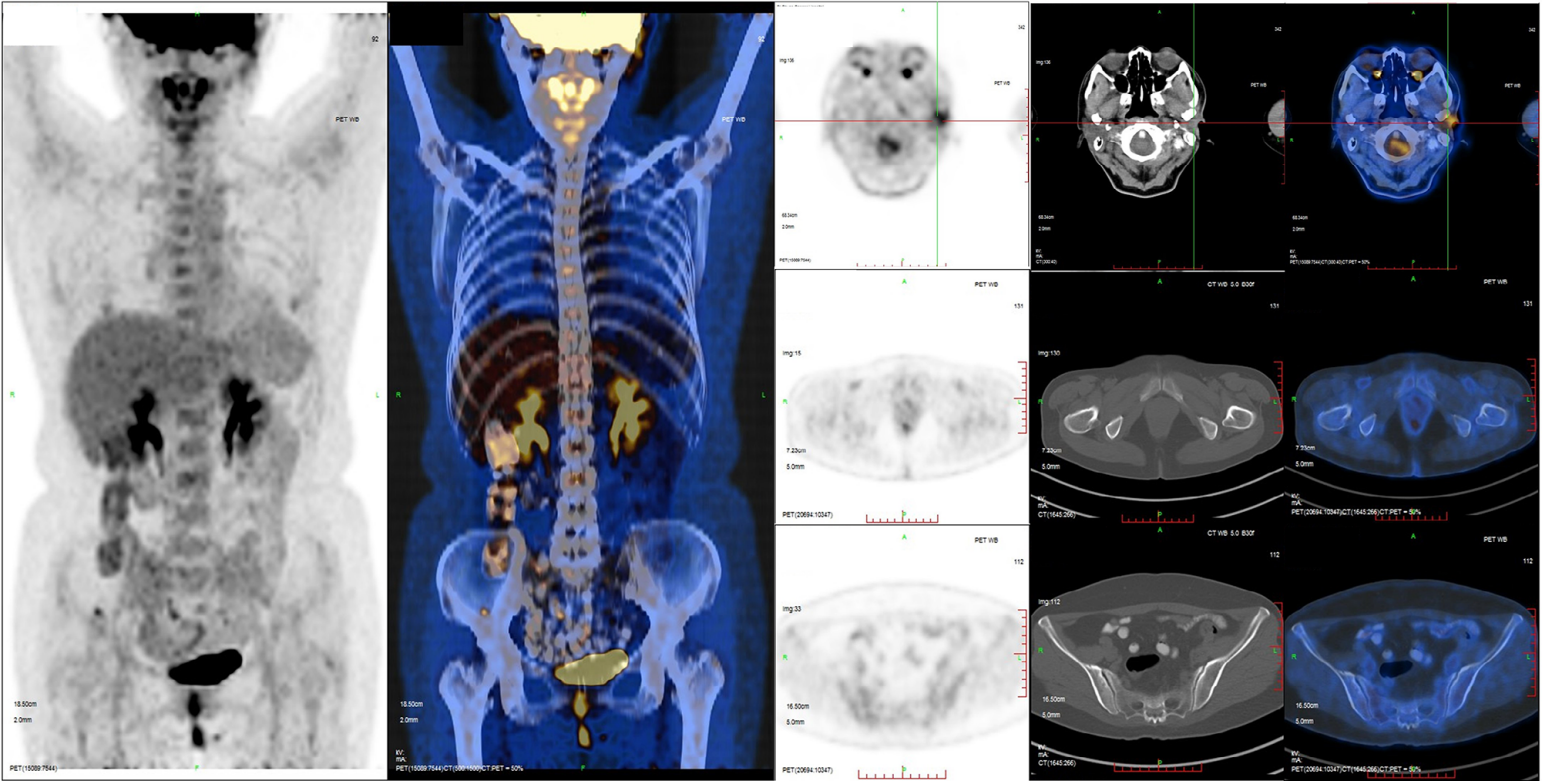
Post‐surgical PET/CT images of the patient.
